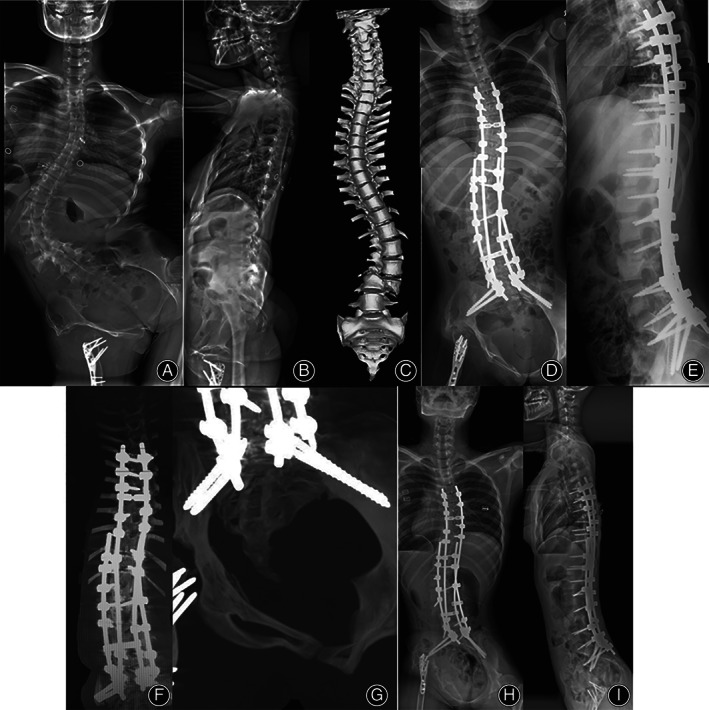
Female, 18 years old, diagnosed with osteopsathyrosis. (A, B) The pre‐operative anteroposterior radiograph showed that Cobb angle was 87.2°, SPO was 51.3° and CB was 42.1 mm. (C) pre‐operative CT scans showed multiple fractures of lower limbs and L3/4 fracture dislocation. (D, E) Postoperative anteroposterior radiograph showed significant correction of kyphoscoliosis and PO, the Cobb angle was 25.4°, SPO was 18.7° and CB was 16.2 mm. (F, G) Post‐operative CT scans showed the placement of bilateral dual S2AI screws. (H, I) At 1‐year follow‐up, the anteroposterior radiograph showed the correction maintained well, the Cobb angle was 27.2°, SPO was 16.1° and CB was 10.3 mm.
